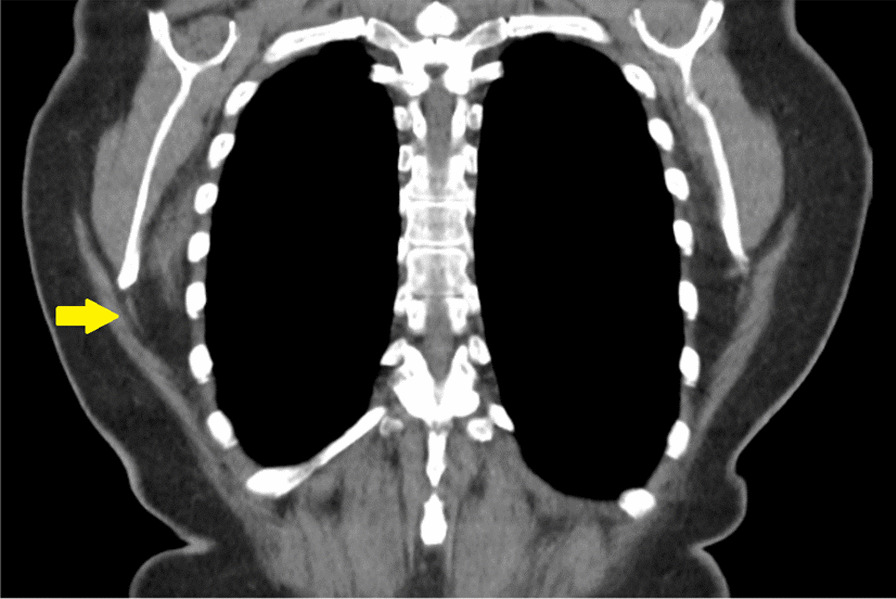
Computed tomography of a case with elastofibrolipoma (yellow indicator)
